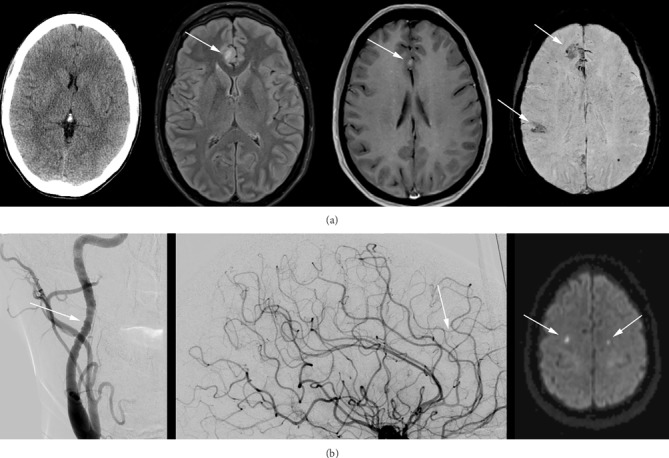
(a) Left to right: computed tomography (CT), fluid-attenuated inversion recovery (FLAIR), T1 post-contrast, and susceptibility-weighted imaging (SWI) 13 days after admission showed minimal findings on CT, but FLAIR hyperintensities and petechial hemorrhages on T1 and SWI images (arrows). These had progressed compared to initial imaging (not shown). (b) Left to right: Carotid and cerebral angiography showing irregular corrugated and beaded appearance of the cervical carotid arteries and subtle intracranial vessel narrowing (arrows), and two new foci of restricted diffusivity on diffusion weighted imaging (DWI) in the bilateral centrum semiovale consistent with interval infarcts (arrows). Note: there was another infarct in the left centrum semiovale on an adjacent slice not shown above.
